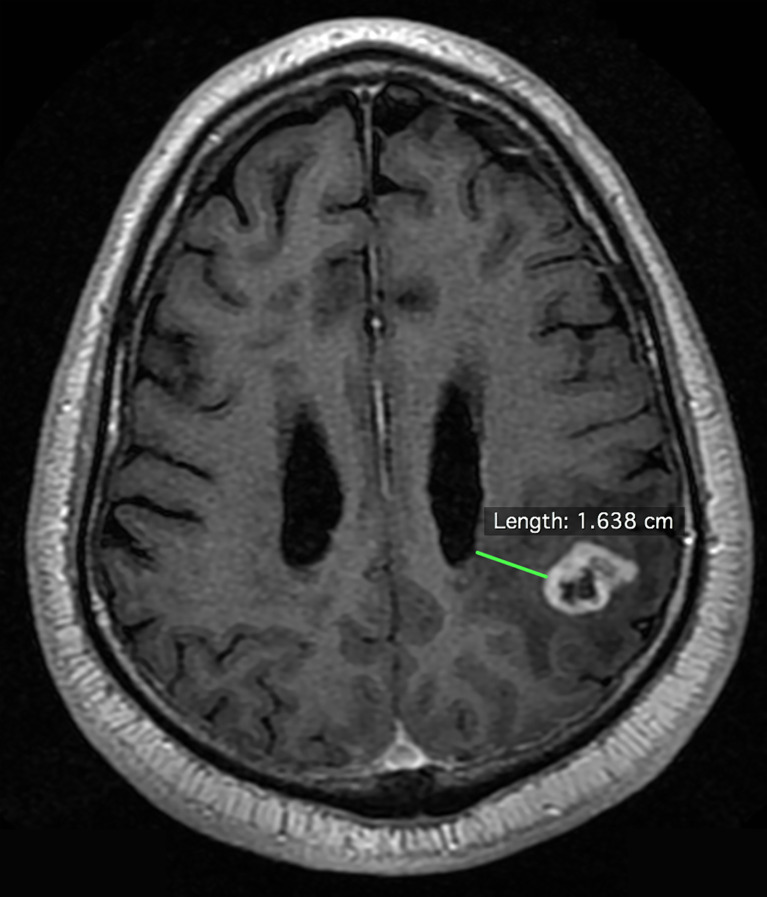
Example measurement of the shortest distance between the enhancing edge of a glioblastoma to the lateral wall of the lateral ventricle on an axial pre-operative gadolinium-enhanced, T1-weighted magnetic resonance image.
